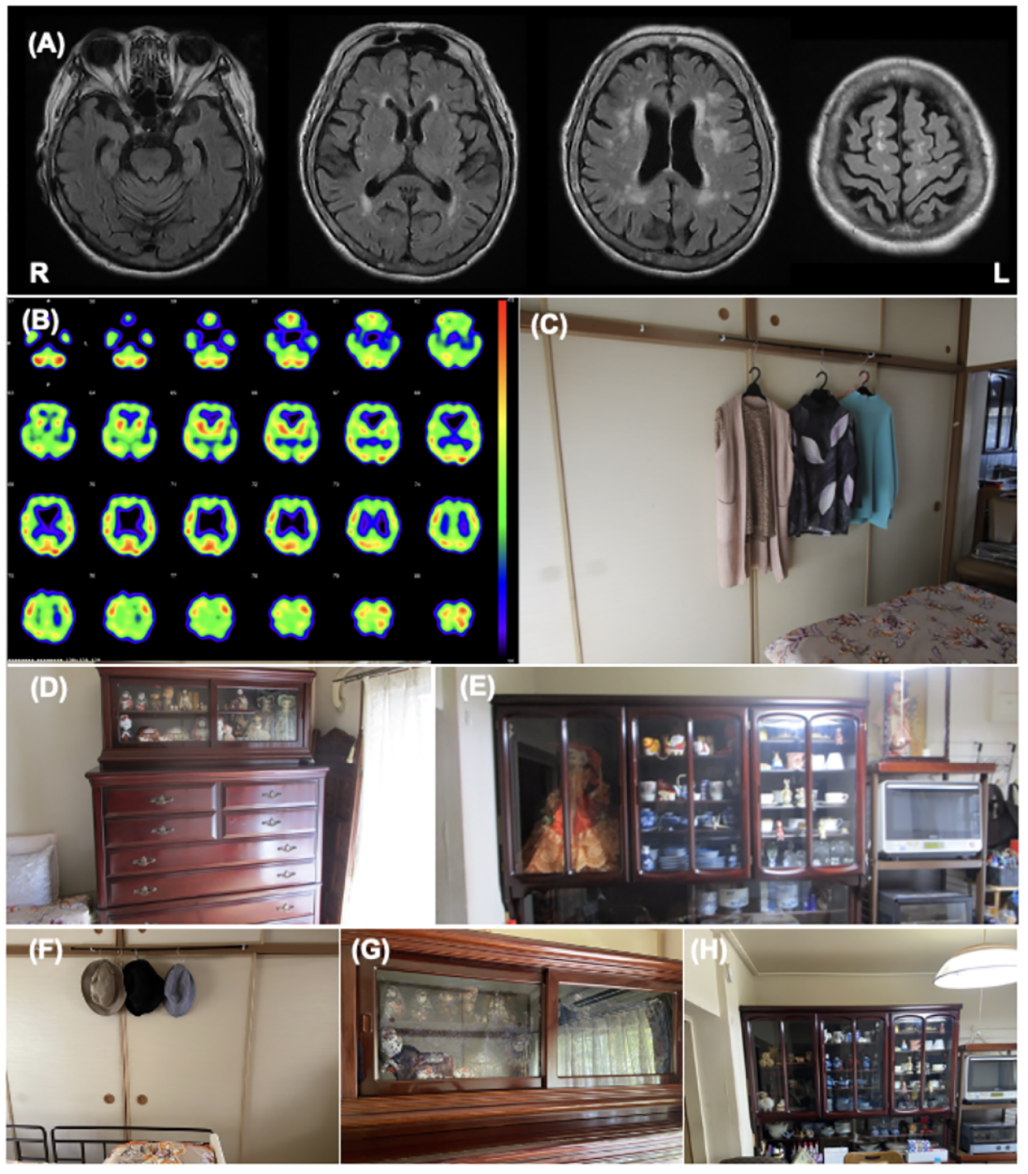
Patient 2 images (A) The cranial MR T2 FLAIR image shows bilateral atrophy of the medial temporal lobe with an extensive high intensity area. (B) I-^123^IMP SPECT shows diffuse reduction of brain perfusion. (C-E) These images show the environmental triggers associated with the visual hallucinations. Clothing on hangers in the bedroom (C), dolls in the bedroom (D), and dolls in the living room (E) were suspected of inducing her visual hallucinations as follows: “Male strangers intruded my home from the balcony”, or “Children looked for something in the chest of drawers”. (F-H) These images show the living environment post intervention. The clothes on hangers were removed (F), the dolls in the bedroom were hidden using blindfold shades (G), and the number of dolls in the living room was reduced (H).
